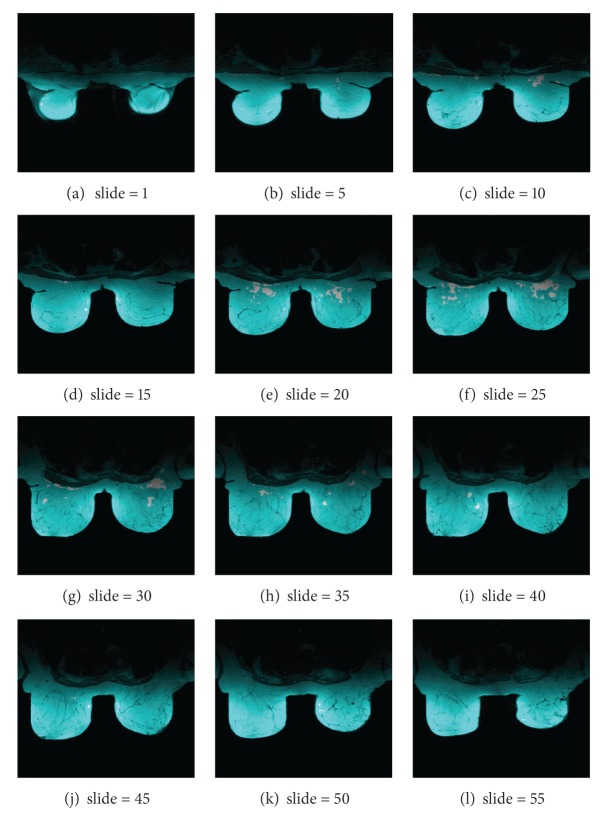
Twelve different slides from the MRI image described in Section 3. The image output from the system is colored for the sake of clarity in recognition. White zones mean high motion density.
